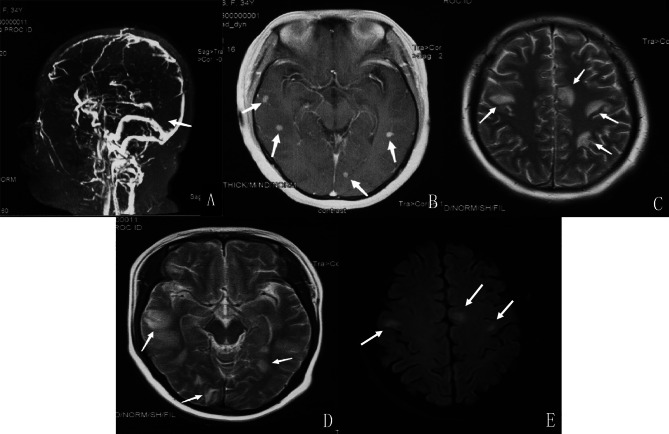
Radiographic findings of the patient in case 1. A: MRV shows partial visualization with thrombosis of the straight sinus; B: T1-weighted MRI of the brain with contrast images showing enhancement of multiple nodules in the temporal and occipital lobes; C, D, and E: MRI revealed infarction of bilateral frontoparietal temporal and left occipital lobes
